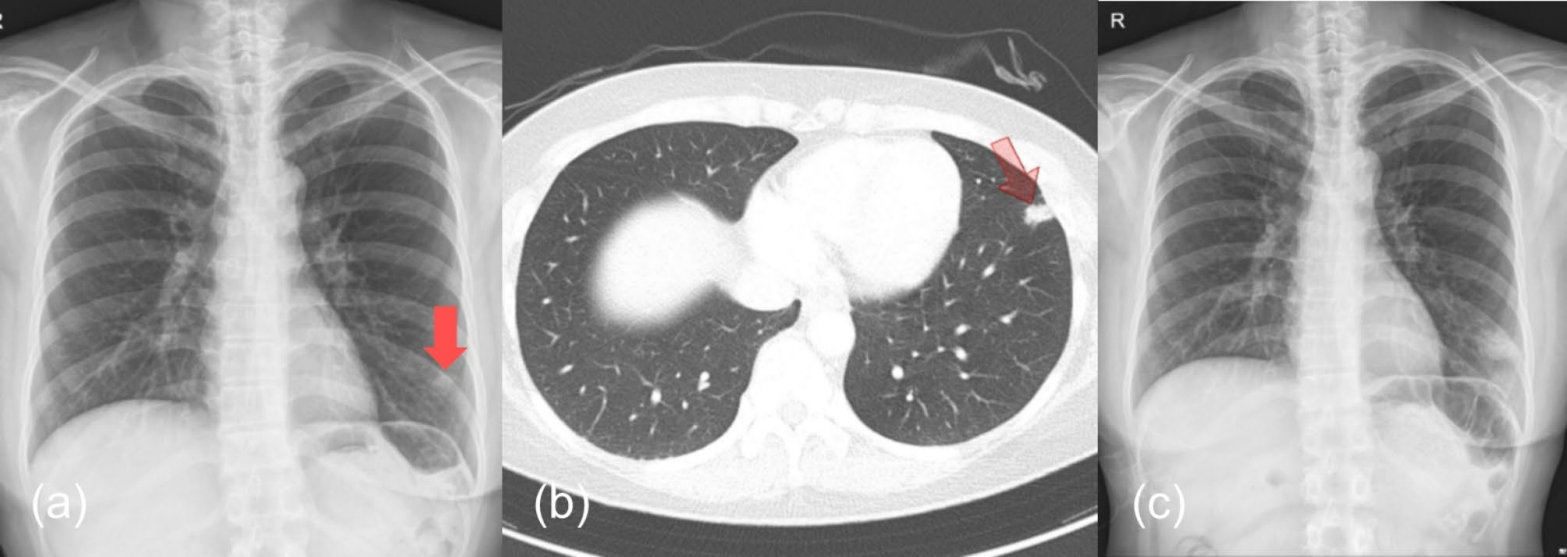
(a) Chest radiograph showing a solitary pulmonary nodule (solid red arrow) in the left lower lung field before cryobiopsy. (b) Chest CT image demonstrating a peripheral pulmonary nodule (hollow red arrow) in the left lower lobe. (c) Increased density in the lower zone of the left lung after cryobiopsy, as shown by chest radiograph
